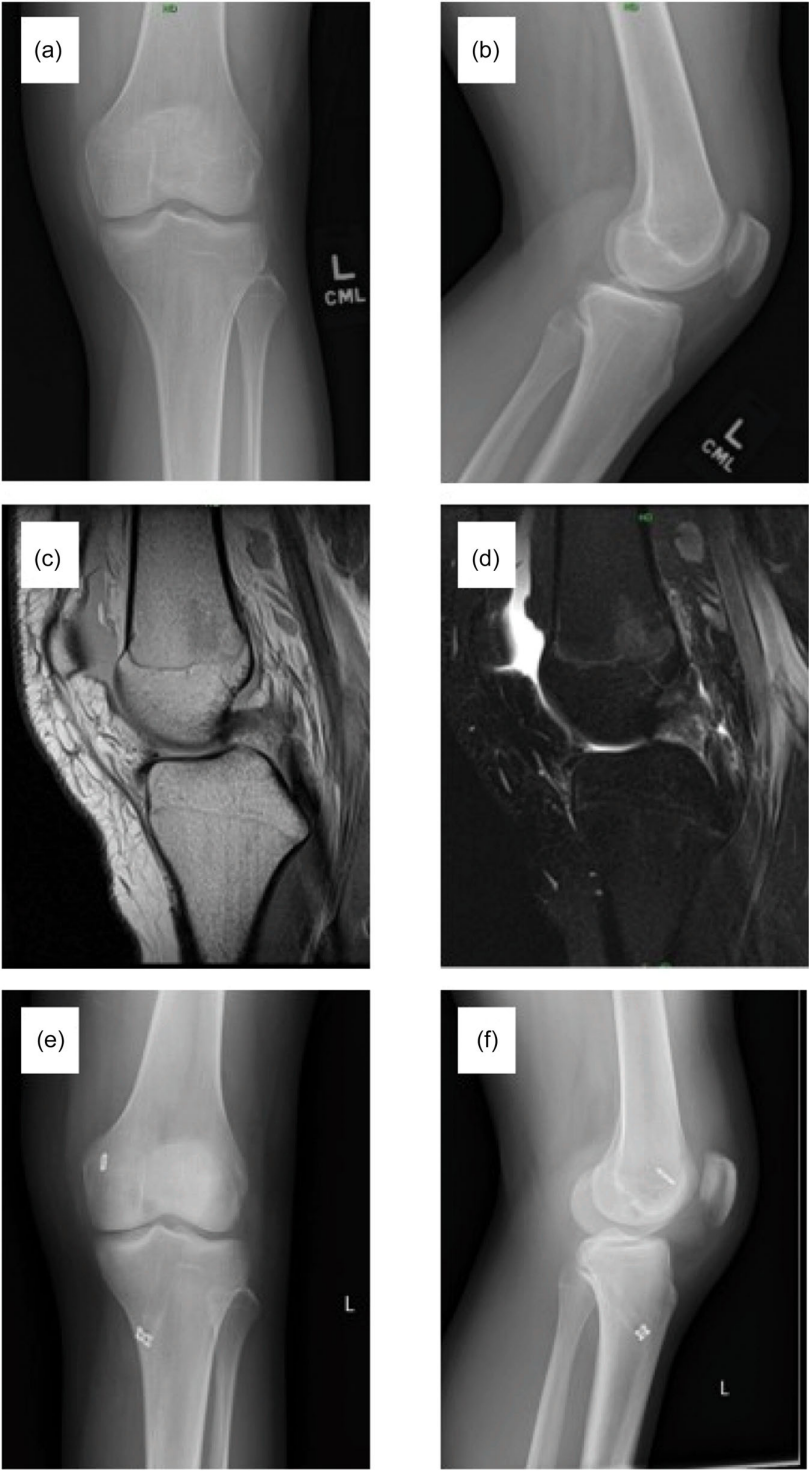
Radiographic imaging of a 17‐year‐old male who sustained a complete PCL tear while skateboarding that was managed surgically with PCL reconstruction using tripled posterior tibial tendon allograft. The patient successfully returned to sports after 9 months of physical therapy and sustained no recurrent injuries at latest follow‐up. (a) Pre‐operative anterior/posterior view x‐ray. (b) Pre‐operative lateral view x‐ray. (c) Sagittal view T1 weighted MRI. (d) Sagittal view T2 weighted MRI. (e) Post‐operative anterior/posterior view x‐ray. (f) Post‐operative lateral view x‐ray. MRI, magnetic resonance imaging; PCL, posterior cruciate ligament.
